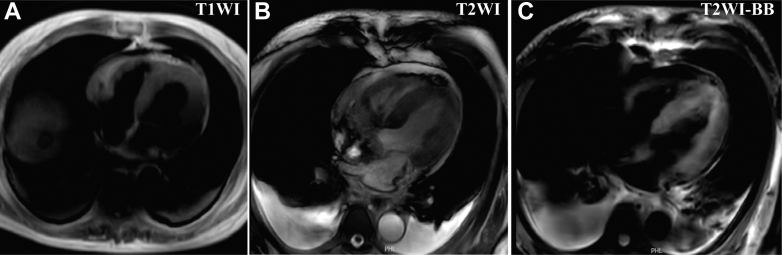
Cardiac Magnetic Resonance Findings Cardiac magnetic resonance performed on day 12 post-admission: (A) T1-weighted imaging (T1WI), (B) T2WI, (C) T2-weighted black blood imaging (T2WI-BB). The images demonstrated pericardial effusion and heterogeneous myocardial thickening. The T2WI-BB sequence reveals increased myocardial signal intensity predominantly in the apical region of the left ventricle.
